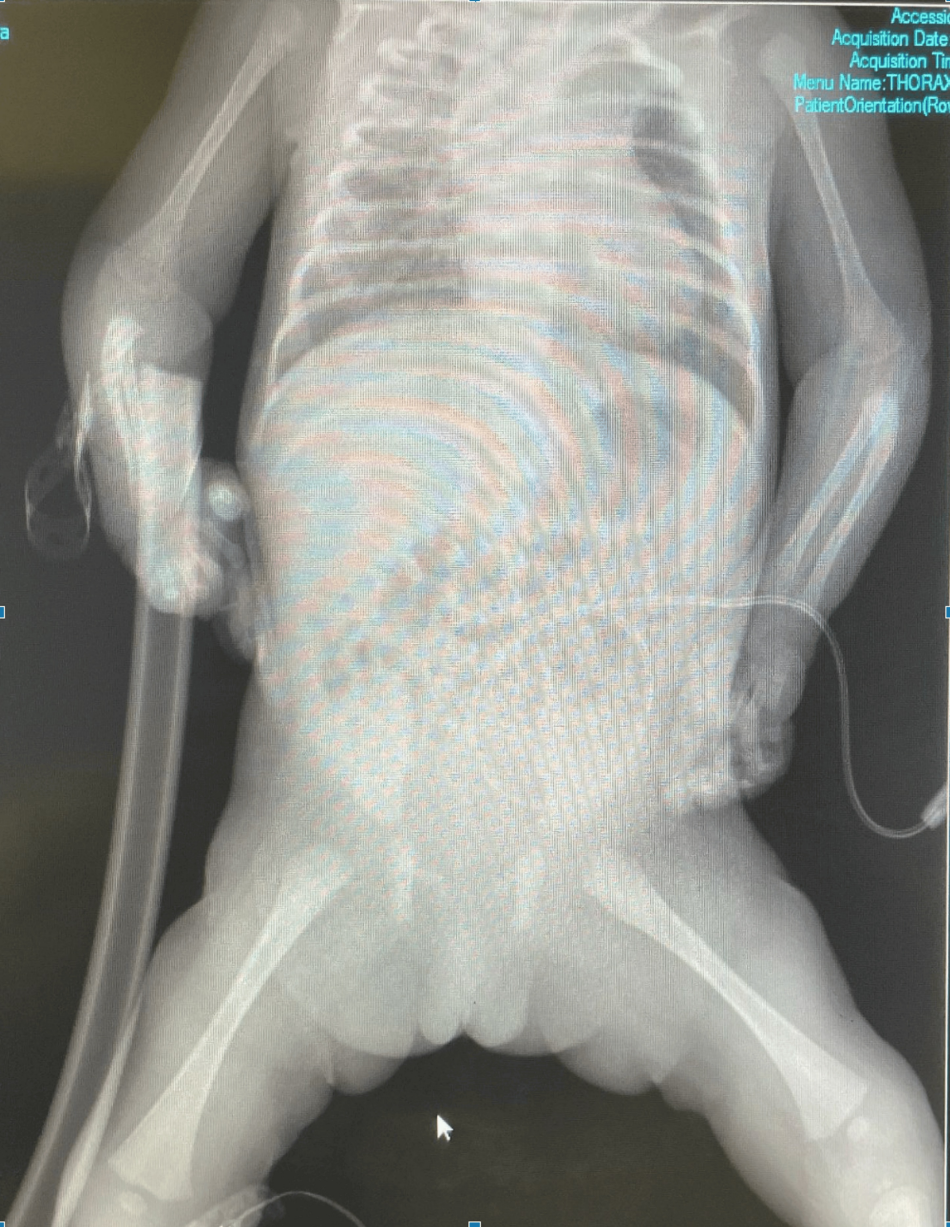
Skeletal radiograph of our patient
